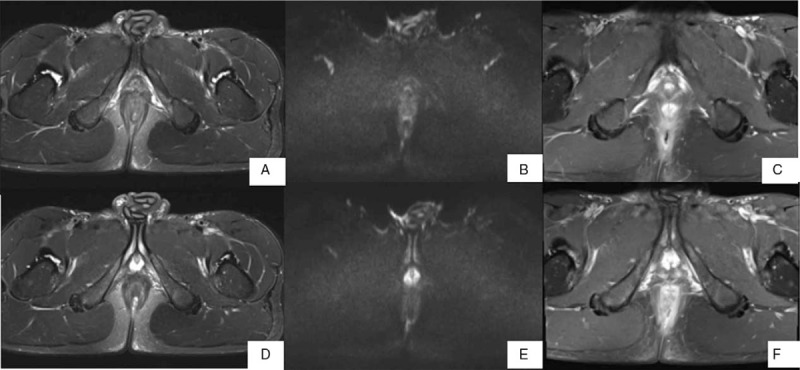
MR images of a 27-year-old man with an intersphincteric perianal fistula. (A, D) Axial fat suppressed T2-weighted imaging (FS T2WI), and (B, E) axial diffusion-weighted imaging (DWI) show the internal orifice (thin arrow) and the fistula tract (thick arrow) respectively. (C, F) The internal orifice is invisible on axial fat suppressed T1-weighted imaging with contrast enhanced (FS T1WI+CE), and the fistula tract (thick arrow) is nebulous because the comparative enhancement of the fistula tract and the inflammatory structure around the fiatula.
